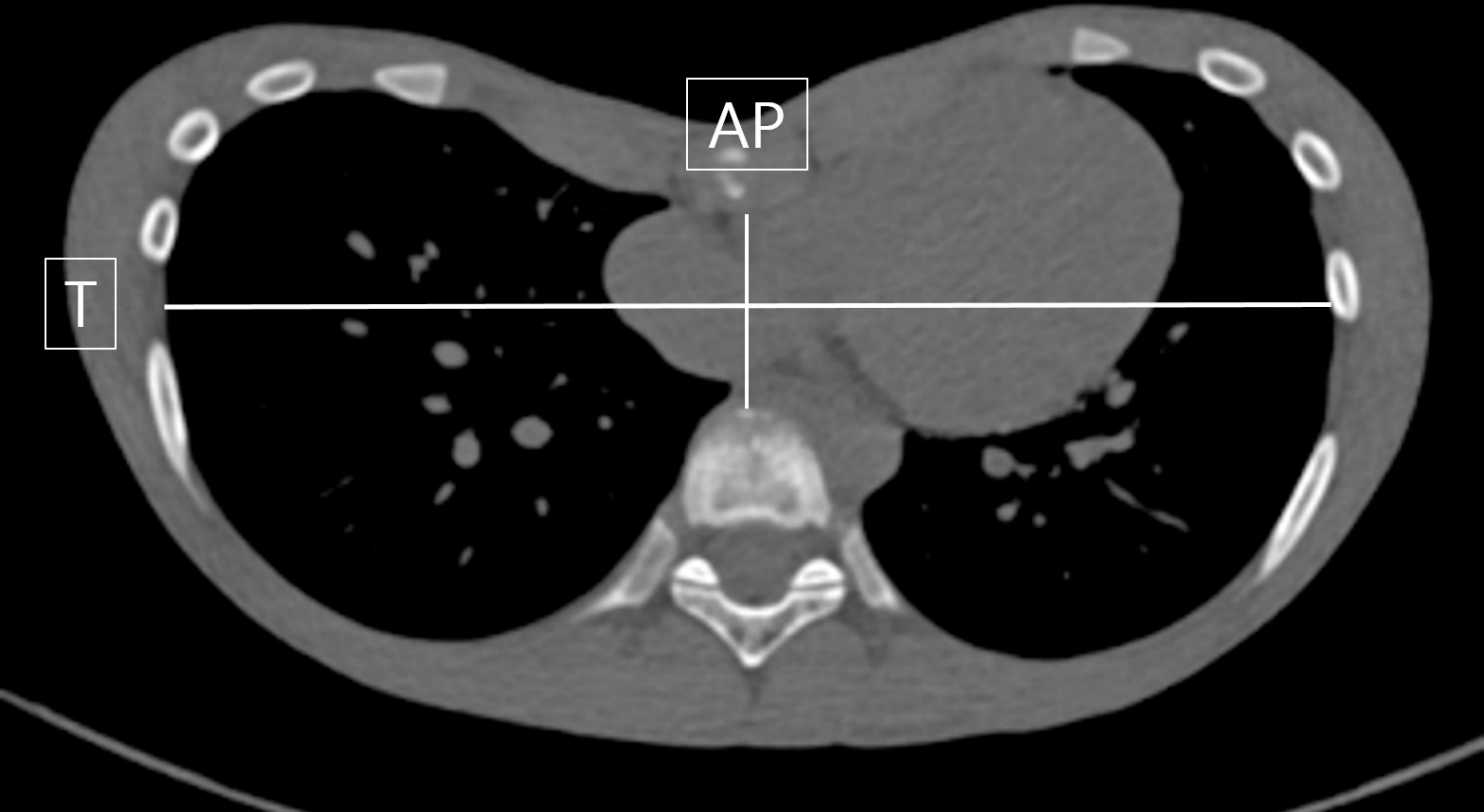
Example of an axial CT image with the measurements required for calculation of the Pectus index (T/AP).
